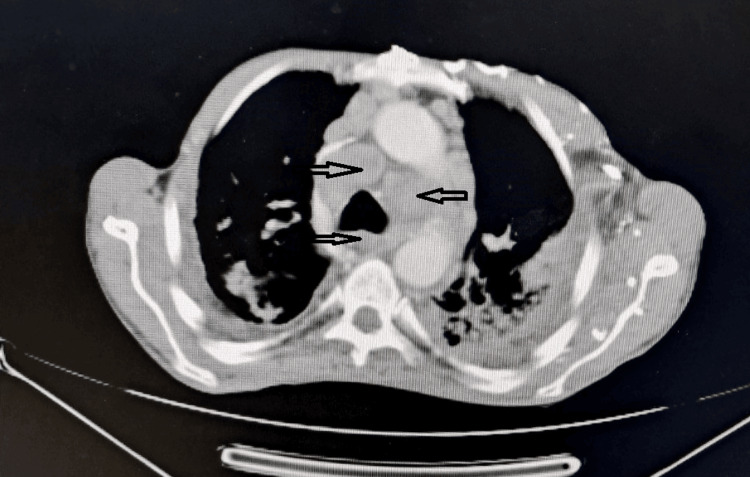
Case 3: Chest CT in axial section, mediastinal window, showing mediastinal lymphadenopathy in a patient who developed pancreatic adenocarcinoma.
